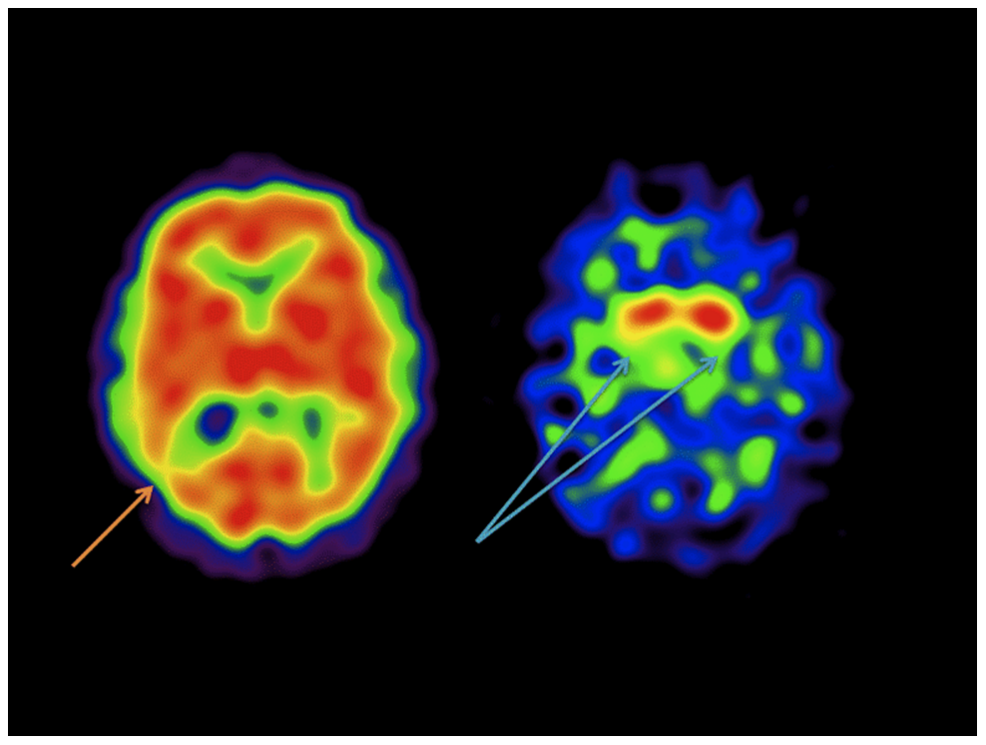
^99m^Tc Exametazime and ^123^I Ioflupane SPECT studies in a 58 year old male subject with a history of a single TBI with clinically presenting with problems with memory, sleep, and vertigo and no clear parkinsonian features. The CBF shows only a mild decrease in the right temporo-parietal region. However, DAT binding is markedly abnormal with preserved uptake primarily in the caudate (R>L) and minimal uptake in the putamen bilaterally.
